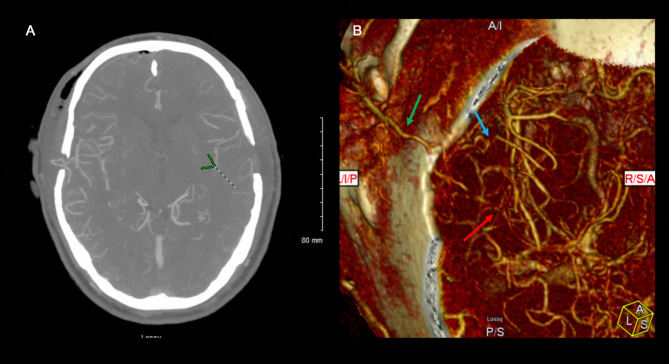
This postoperative CTA demonstrates patency of bilateral STA-MCA bypasses filling both MCA territories robustly. The left sided STA-MCA double barrel bypass is patent (green arrow – main STA trunk; blue arrow – frontal STA to frontal M4; red arrow – parietal STA to temporal M4).
